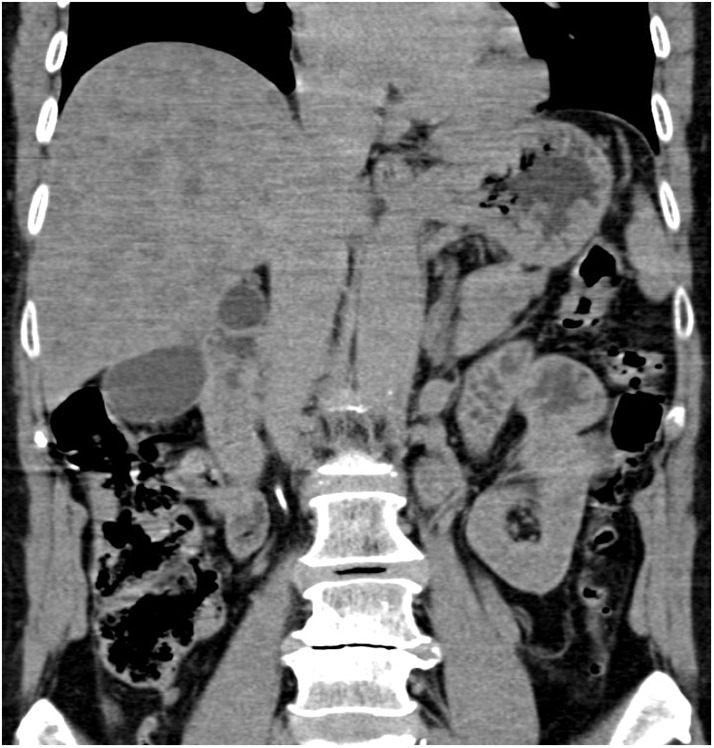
Abdominal computed tomography, non-enhanced coronary scan. Markedly inhomogenous liver with map-like hyperdense areas and multiple hypodense focal changes.
